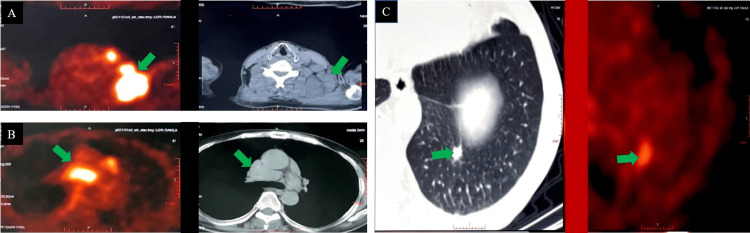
Panel A: PET-CT highlights area showed increased FDG uptake in the cervical lymph nodes; panel B: increased FDG uptake in the thoracic lymph nodes; panel C: increased FDG uptake in the posterior basal segment of the lower lobe of the left lung. PET-CT, positron emission tomography-computed tomography; FDG, fluorodeoxyglucose
